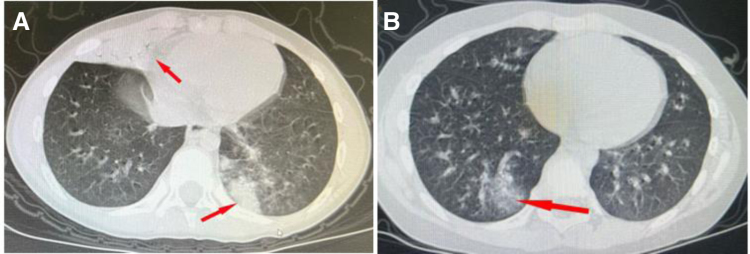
(A) Case 1: Chest CT of the 5-year-old girl showing recurrent right lower lobe inflammation and consolidation. (B) Case 2: Chest CT of the 5-year-old boy showing inflammatory changes with consolidation in both lungs. CT = computed tomography.
